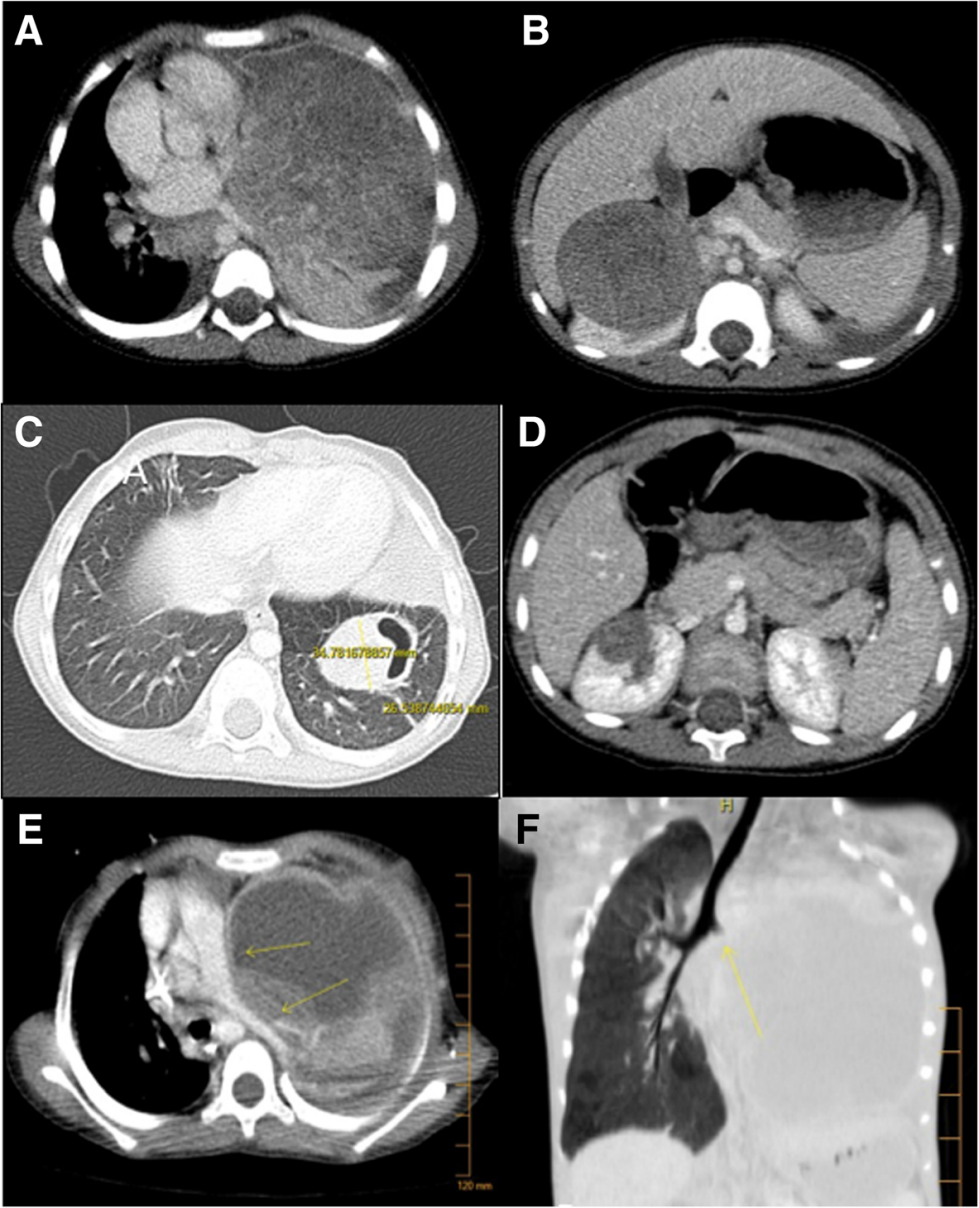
CT scan evolution through pre-operative chemotherapy. a, b Pulmonary and renal lesions at diagnosis. c, d Good response after WT chemotherapy. e, f Dramatic pre-operative evolution after modification of the chemotherapy drugs, which led to emergency pneumonectomy due to acute symptoms. Arrows indicate the narrowing pulmonary artery
